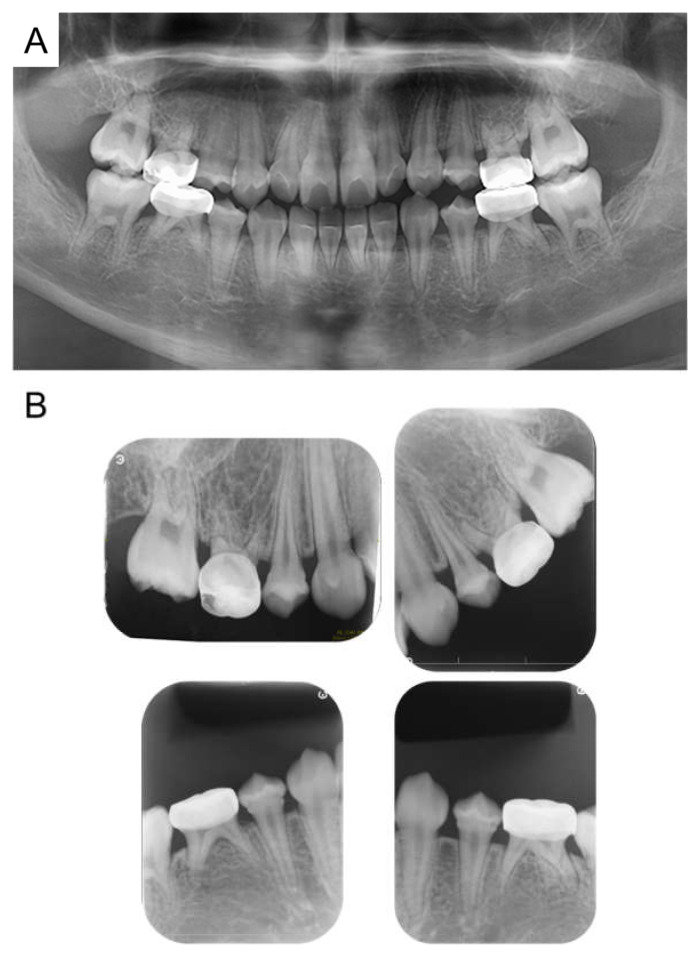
Images at the age of 11 years and 6 months. (A) Panoramic radiograph. (B) Periapical radiographs.
